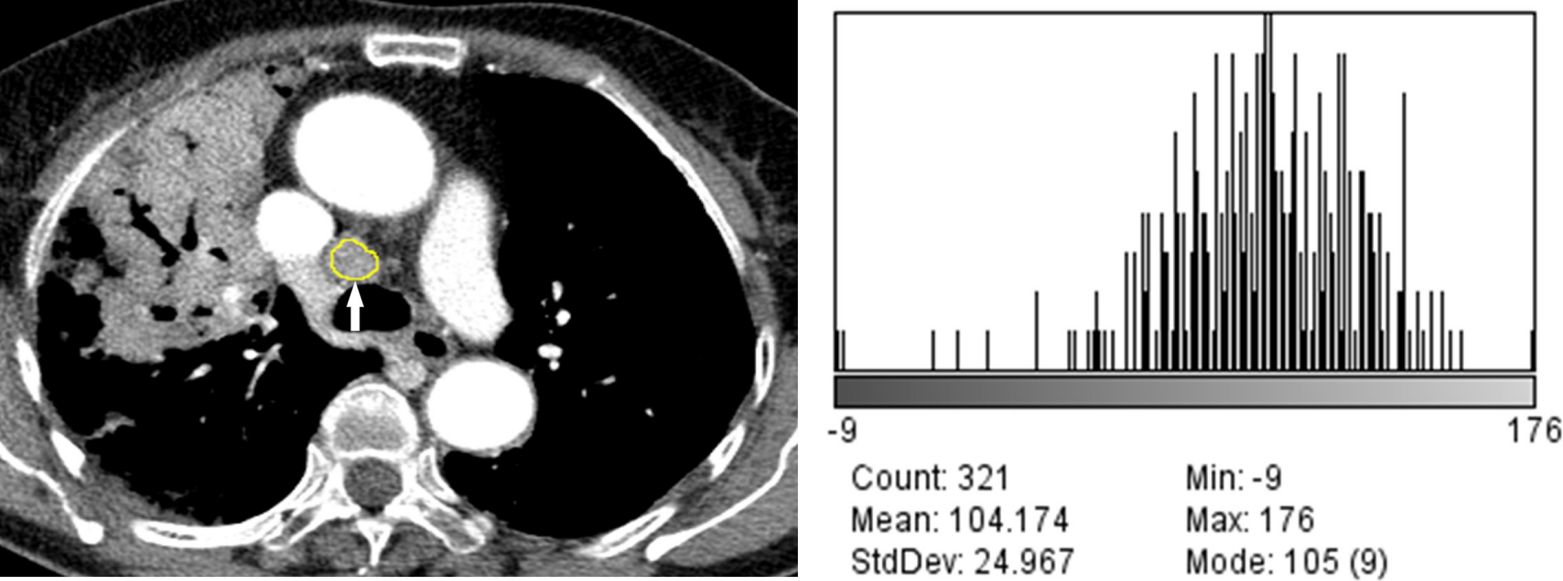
76-year-old woman with tuberculous lymphadenopathy with lung involvement. (A) Mediastinal window image of contrast-enhanced chest CT scan shows a segmented right lower paratracheal lymph node (arrow). (B) Histogram plot shows the CT data profile of the segmented lymph node (skewness, -1.14; kurtosis, 2.86).
